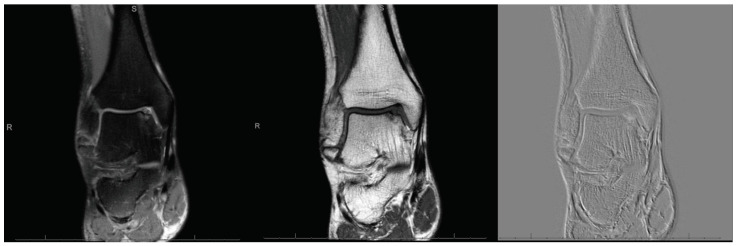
MRI evidencing a focal defect of the medial talar shoulder.
